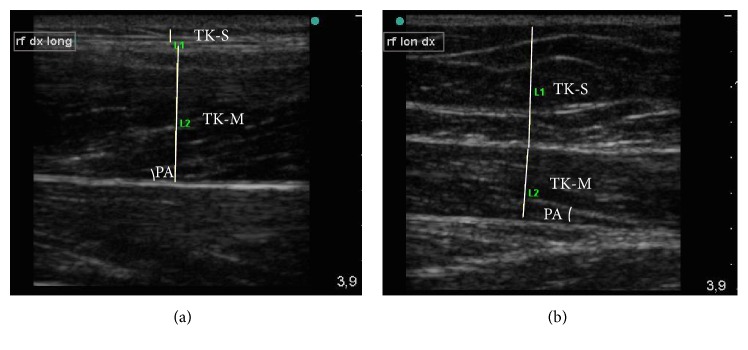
Examples of ultrasound images of the rectus femoris. Comparative evaluation of the muscle rectus femoris (RF) in the sagittal view. (a) Ultrasound image of the RF from a typically developed subject. (b) Ultrasound image from a subject with Down syndrome. TK-S, subcutaneous layer thickness (L1); TK-M, muscle thickness (L2); PA, pennation angle between the fascia and the muscle fascicle.
